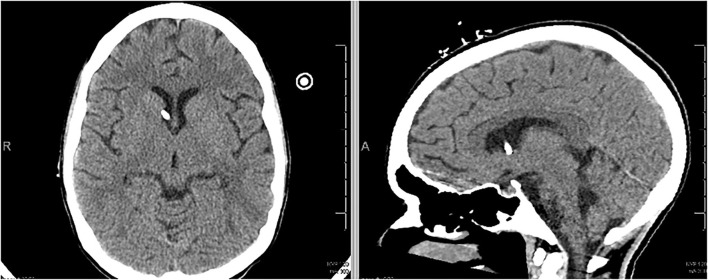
Non-contrast CT scan demonstrating improved cerebral edema on day of EVD removal.
